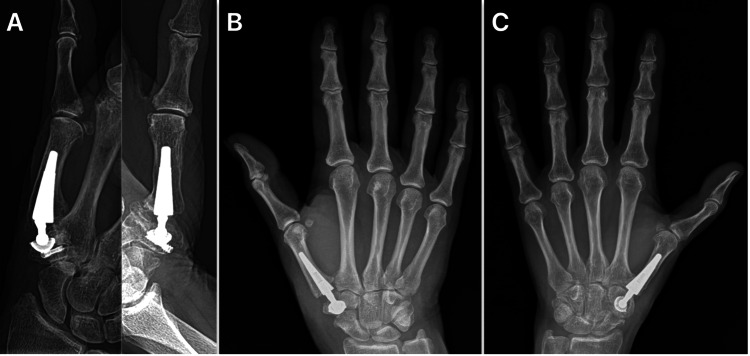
(A) Postoperative radiographic imaging in anterior-to-posterior (AP) and lateral projection of the intraoperative trapezium fracture. (B) Radiographic imaging in AP projection of the postoperative resorption of the trapezium. (C) Radiographic imaging in AP projection of the postoperative fracture of the trapezium following a fall.
